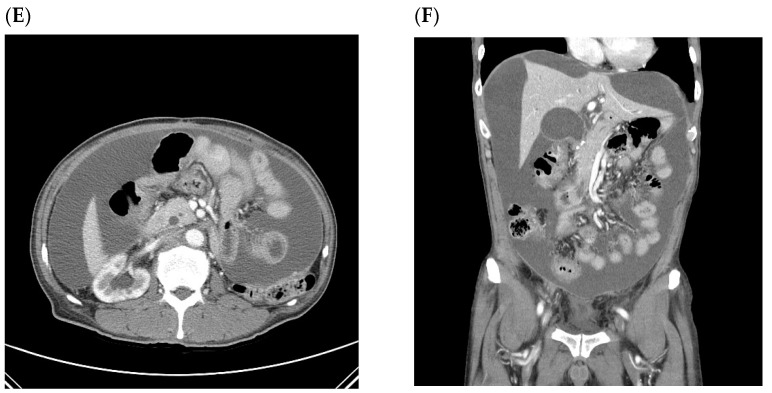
Typical ascites imaging in CT: (A) benign small pelvic ascites; (B) malignant small pelvic ascites with peritoneal thickening; (C) benign moderate ascites in perisplenic area (left abdomen); (D) malignant moderate ascites; and (E,F) alignant large ascites. Yellow stars indicate ascites.
